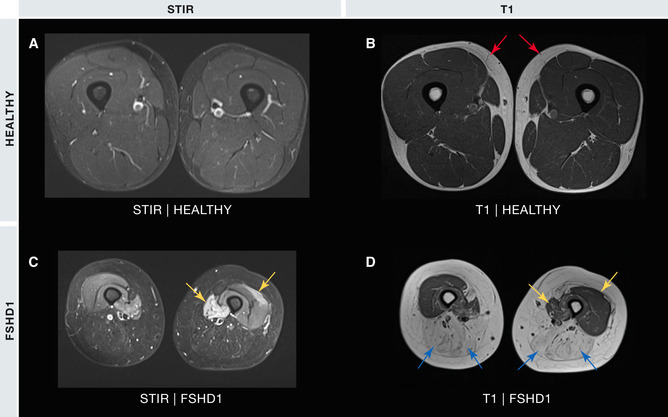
Macroscopic muscle pathology in FSHD MRI images from the thighs of a healthy 24‐year‐old male (A and B) and a 31‐year‐old female with FSHD1 (C and D), where white areas denote high signal intensity of the corresponding sequence. STIR images reveal a uniform signal across the thigh muscles of the healthy male (A). STIR images of the FSHD1 patient (C) display bright signals revealing muscle oedema/inflammation in parts of the vastus medialis and vastus lateralis (C – yellow arrows). The T1‐weighted image of the healthy muscle is uniformly low (B), contrasting with the high (white) signal from the subcutaneous fat (B – red arrows). Muscles of the posterior thigh of the FSHD1 patient (D) have a bright appearance using T1, indicative of fat replacement, including the semitendinosus, semimembranosus, gracilis adductor magnus and biceps femoris (D – blue arrows). The T1 signal remains dark in those portions of the vastus medialis and vastus lateralis of the FSHD1 patient that were bright with STIR imaging (compare C and D – yellow arrows), signifying that fat replacement has not occurred. Published with permission of Giorgio Tasca.
